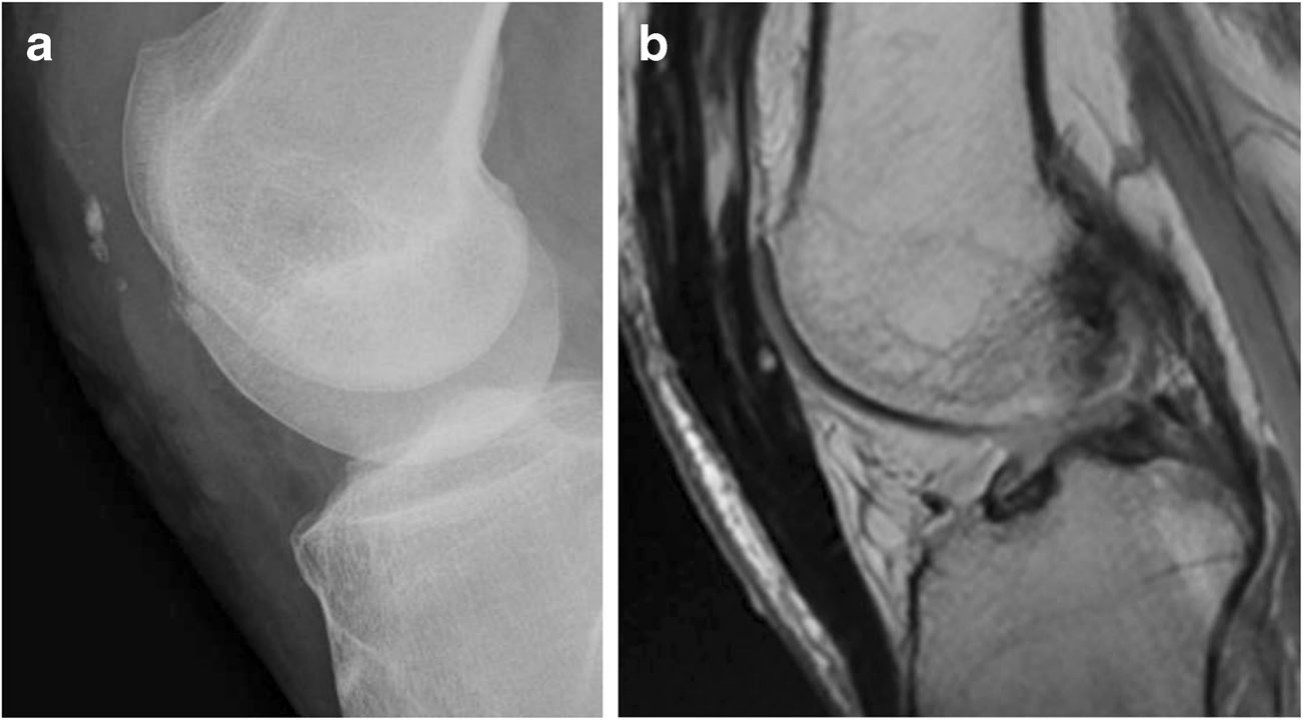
Example of total patellectomy. (a) Lateral knee radiograph and (b) sagittal proton density MR image of the knee after total patellectomy as a result of surgery following a severely comminuted fracture
